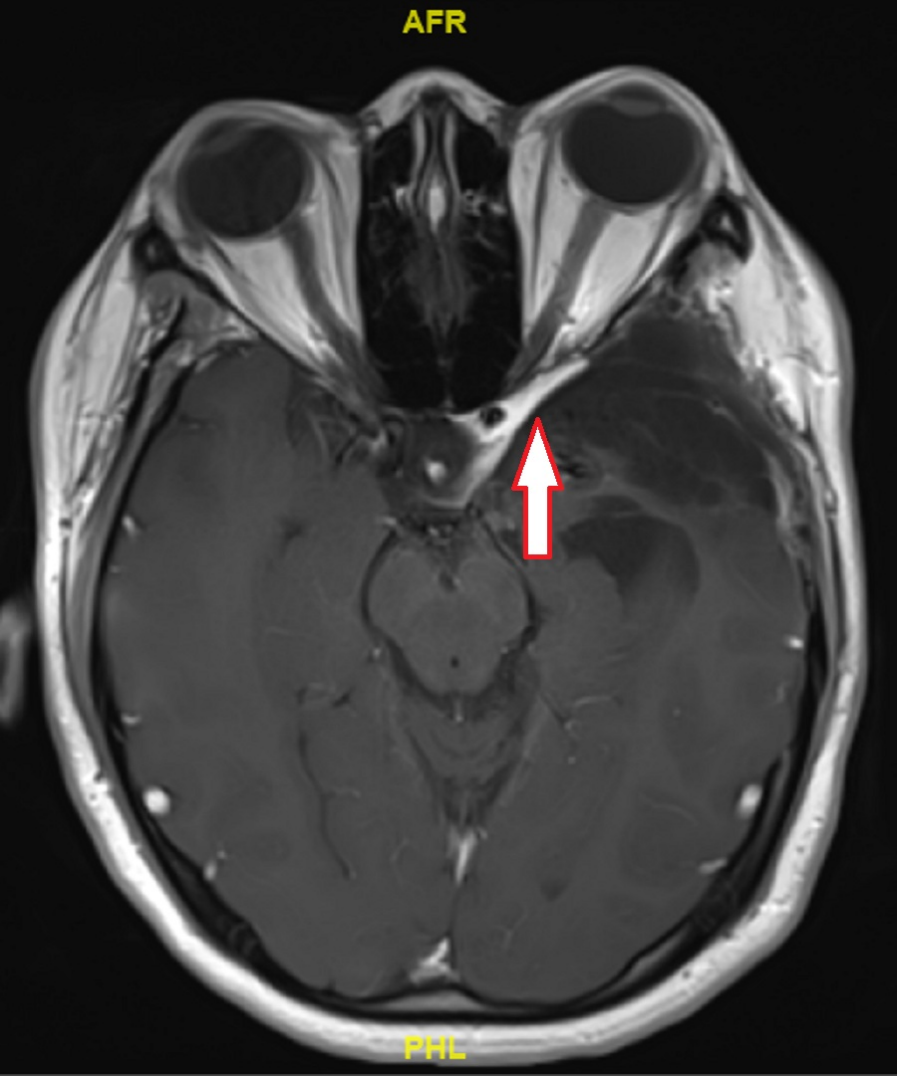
Eighteen months post-Radiation Therapy T1 Contrast-enhanced Magnetic Resonance Imaging Axial view of tumour, demonstrating reduction in size when compared with the immediate post-surgery scan (arrow)
